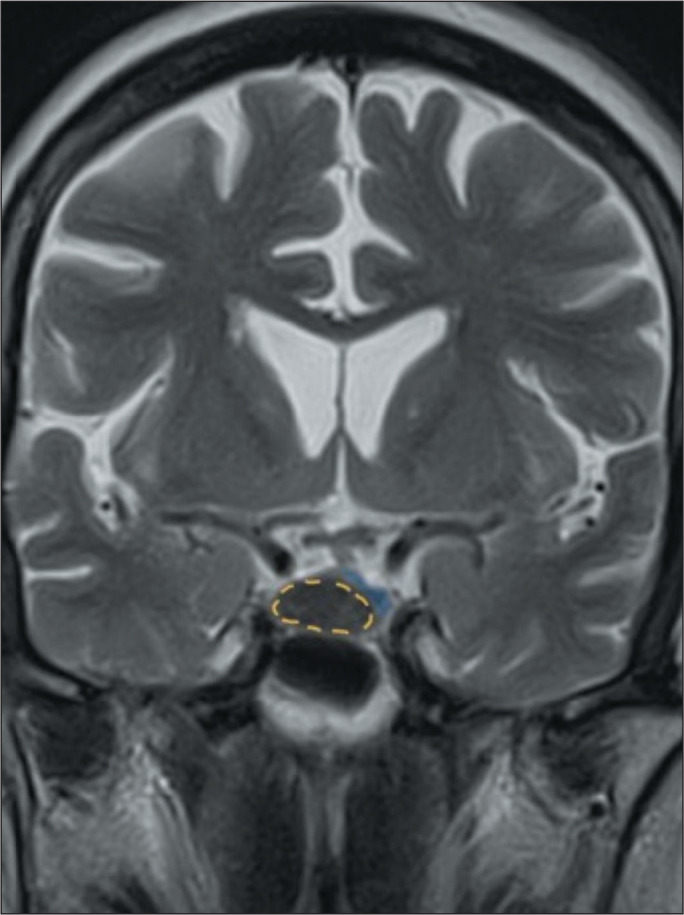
MRI of a Rathke’s cleft cyst in a 63-year-old, asymptomatic patient.
